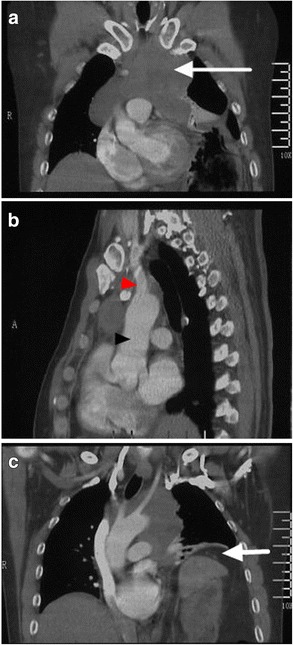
CT scan: Coronal and Sagittal reconstruction (a, b) showed an irregular mass in the anterior mediastinum, about 6.8 × 9.5 cm (the long arrows), surrounding the ascending aorta (the black arrowhead), and brachiocephalic trunk (the red arrowhead). Coronal reconstruction (c) showed elevated left diaphragm, twisted stomach, spleen, parts of bowels and gastric distension (the short arrow)
